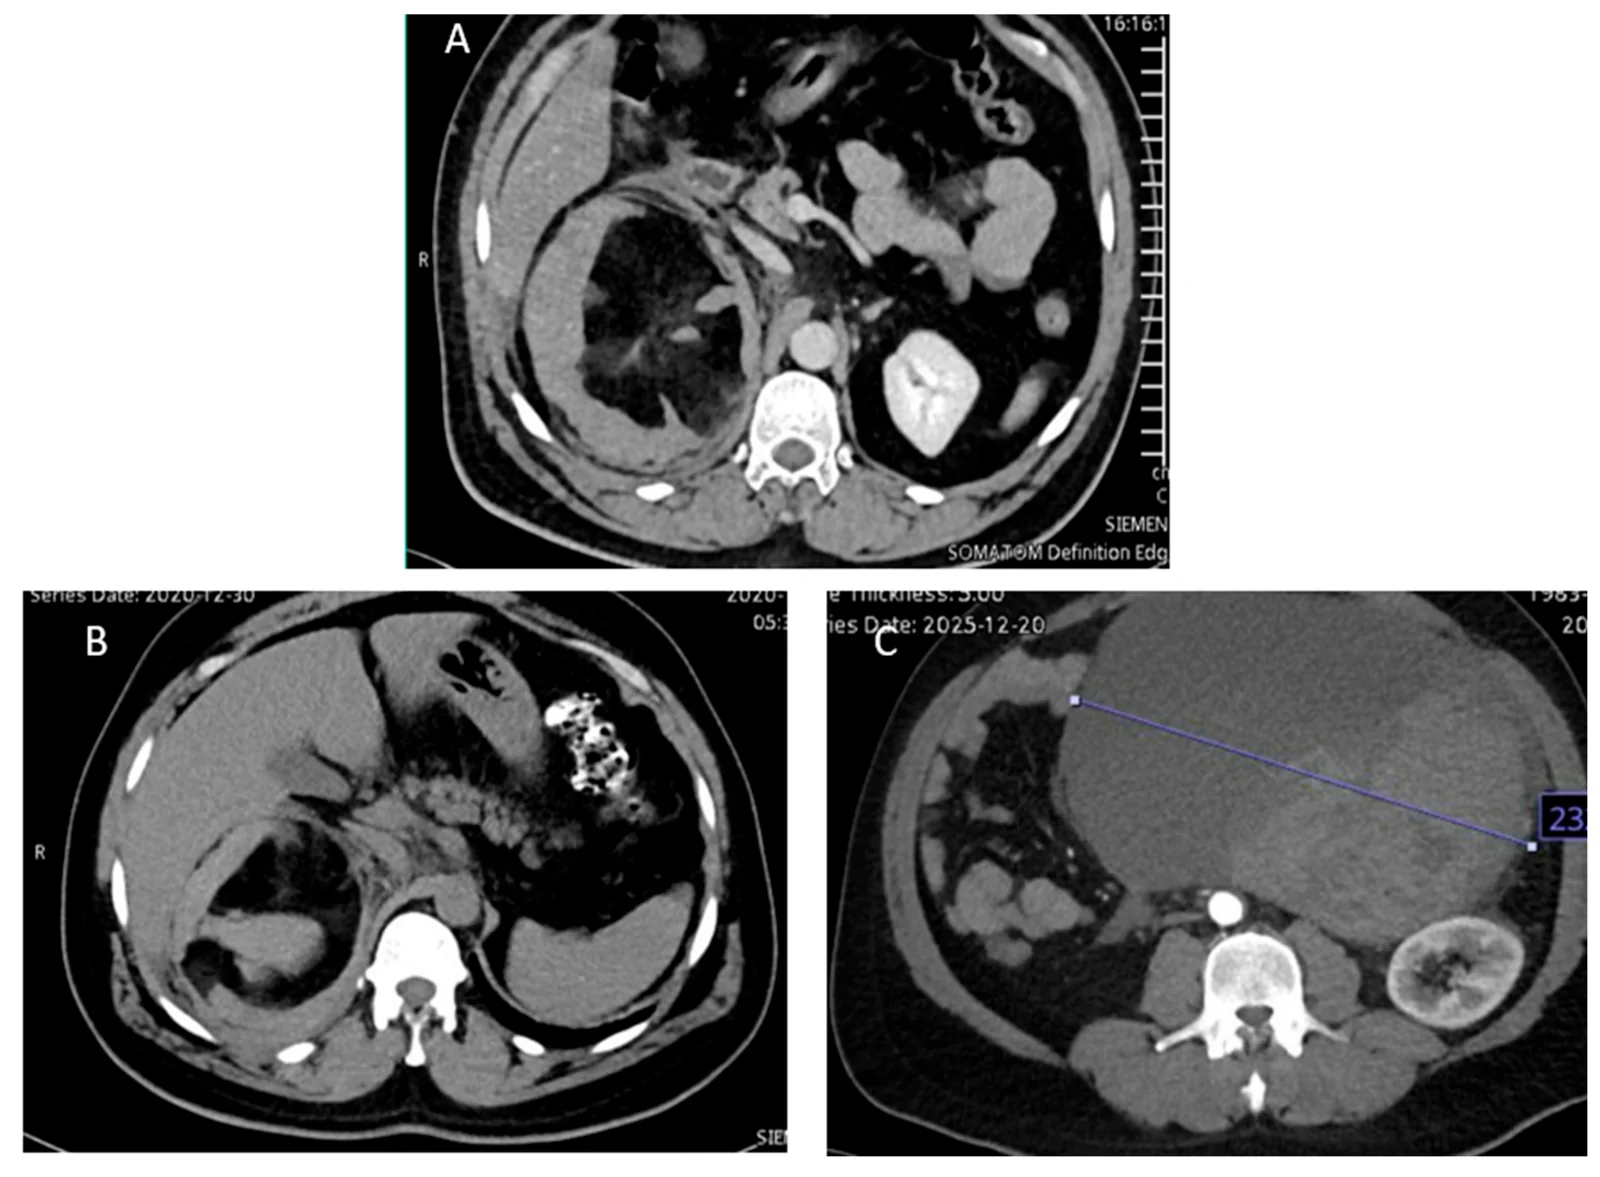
(A): CT abdomen with contrast: a large 12 × 11 × 12 cm heterogenous encapsulated round to avoid a mixed-density lesion in the right suprarenal region with mass effect on right kidney, likely representing a large right adrenal myelolipoma complicated by hemorrhage (Case 11). (B): CT abdomen and pelvis with contrast: Large low attenuation lesion seen in the right suprarenal region, likely originating from the right adrenal gland measuring 10 × 8.5 × 12 cm; features are suggestive of a large right adrenal myelolipoma, with areas of higher density suggesting rupture/hemorrhage (Case:
